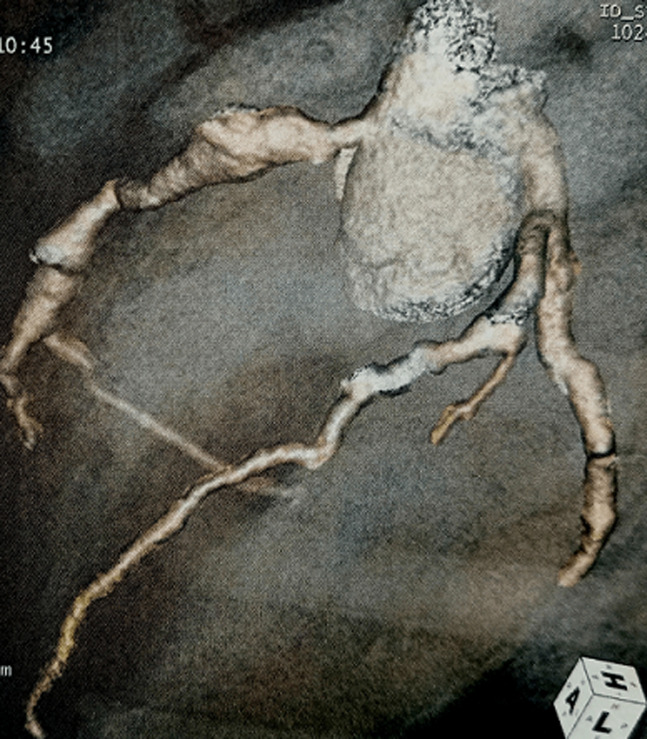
coronary CT angiography revealed multiple coronary aneurysms involving all 3 major coronary arteries
